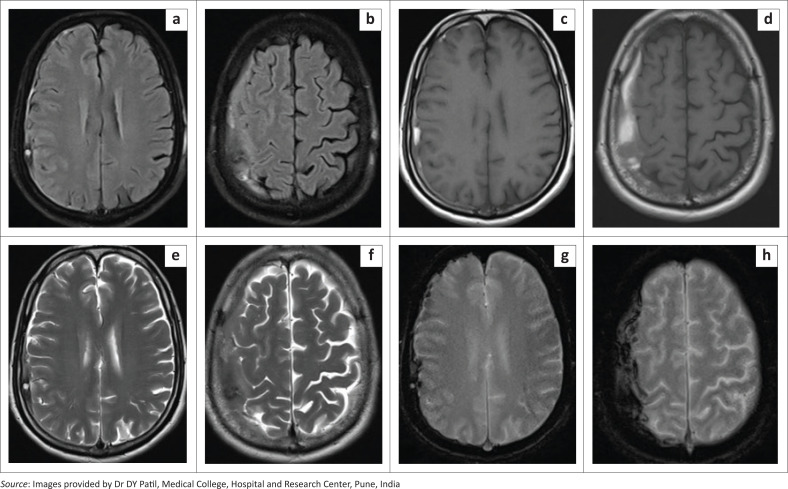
[Case 4]: Brain MRI in a 55-year-old male patient with dengue fever showing a crescent-shaped extra-axial collection overlying the right cerebral convexity, appearing hyperintense on FLAIR (a, b) and T1-weighted (c, d) images and of mixed signal intensity on T2-weighted images (e, f) with blooming on GRE images (g, h), suggestive of an acute to subacute subdural haematoma.
